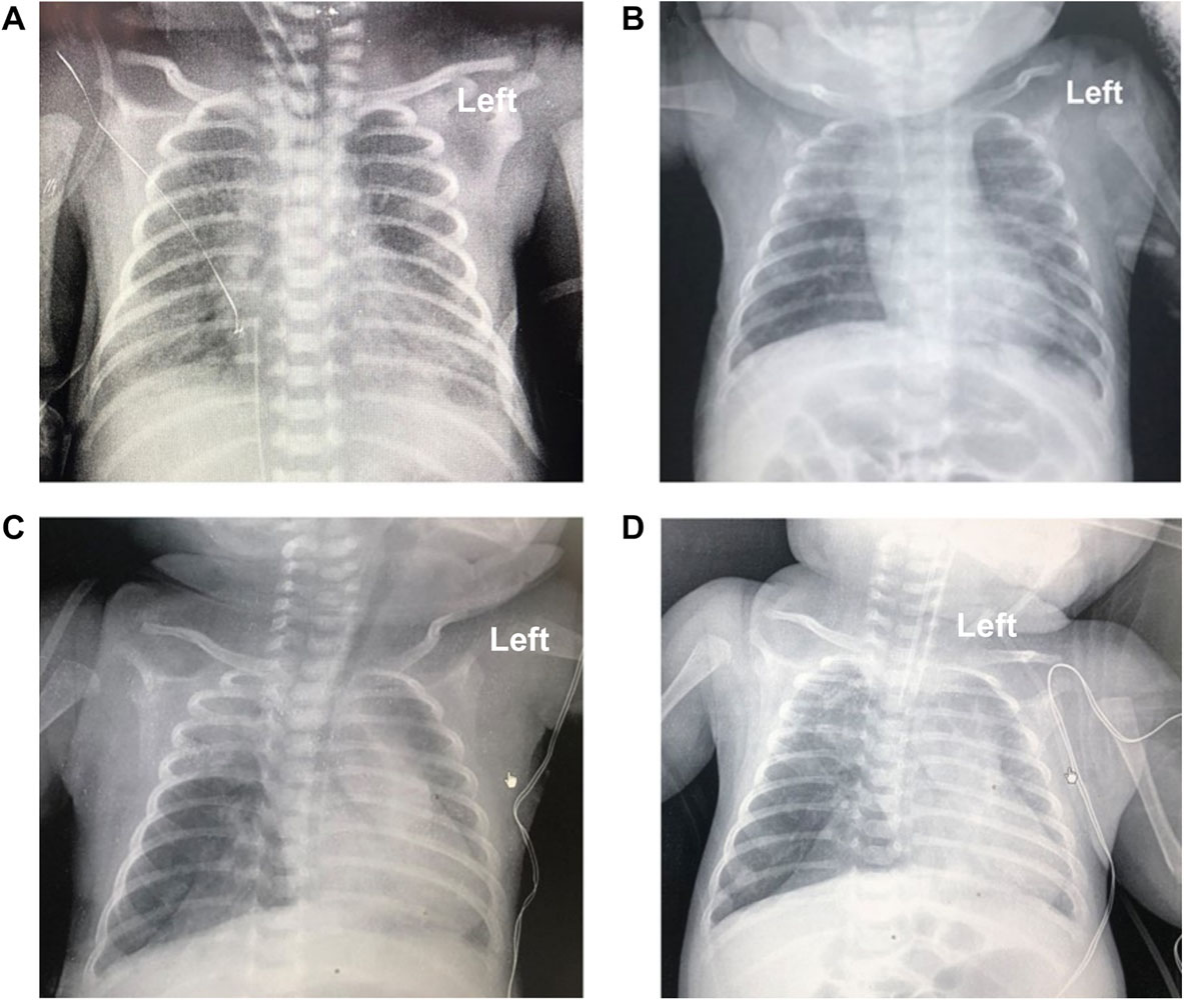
Chest radiography at D1, D10, D55, and D61. Chest X-ray revealed gradual progression of the mediastinal hernia
